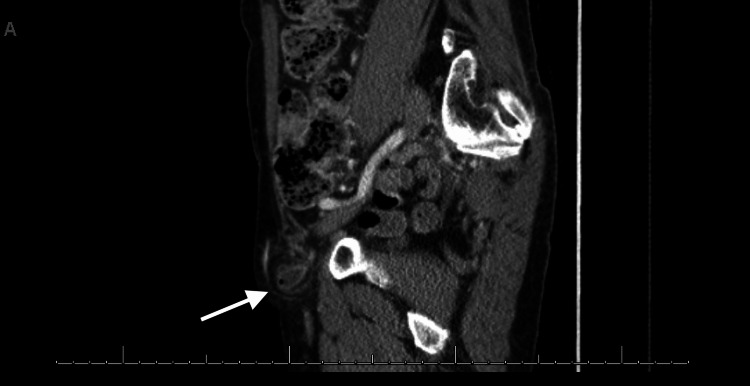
Contrast-enhanced computed tomography of the abdomen and pelvis Sagittal view showing the right femoral hernia with abrupt caliber change of the distal appendix as it enters the hernia sac (white solid arrow). Small amount of reactive free fluid within the hernia sac with associated acute appendicitis secondary to proximal obstruction.
